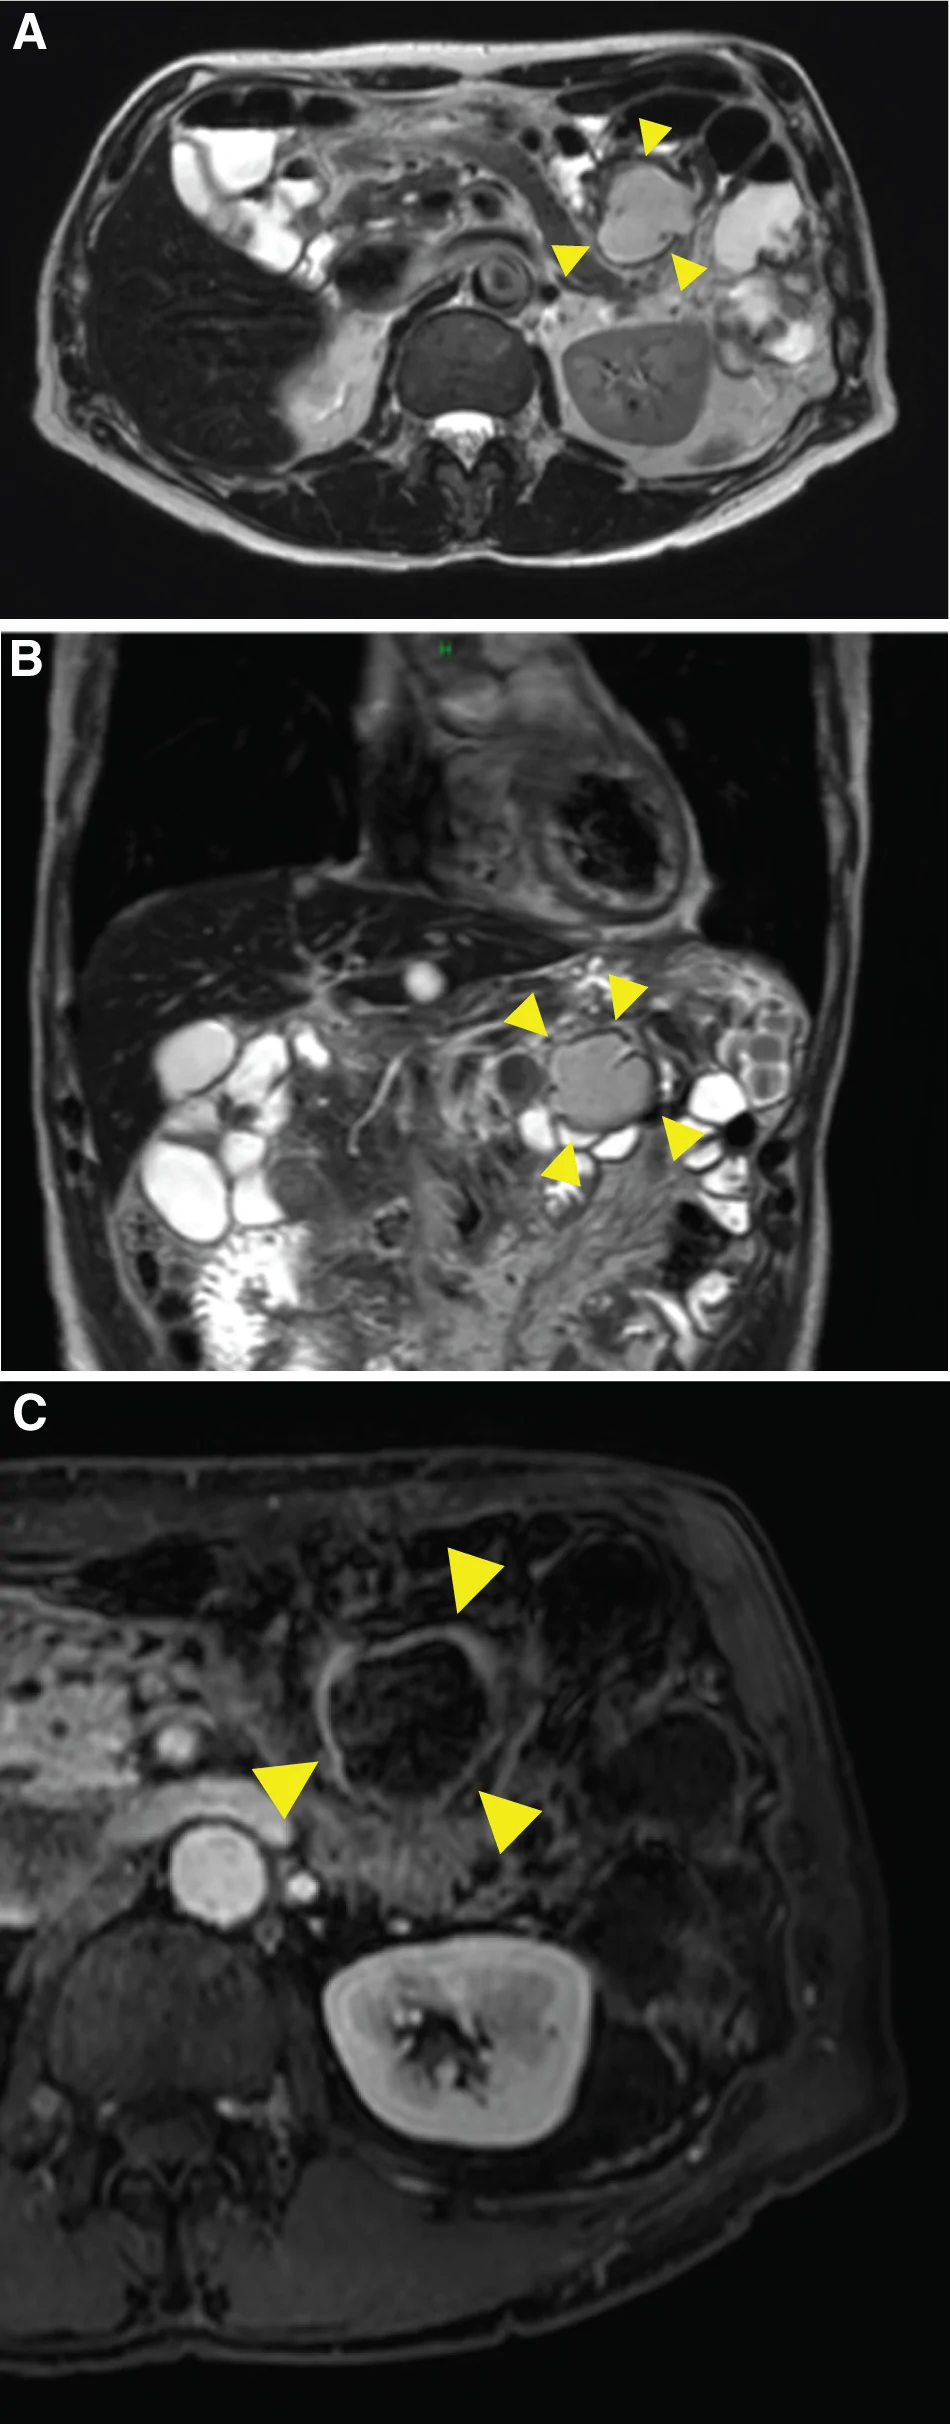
MRI findings. (A, B) A high-signal-intensity mass on T2-weighted imaging (yellow arrowheads). (C) A low signal intensity on fat-suppressed T2-weighted imaging with contrast enhancement overlying the lipoma (yellow arrowheads).
